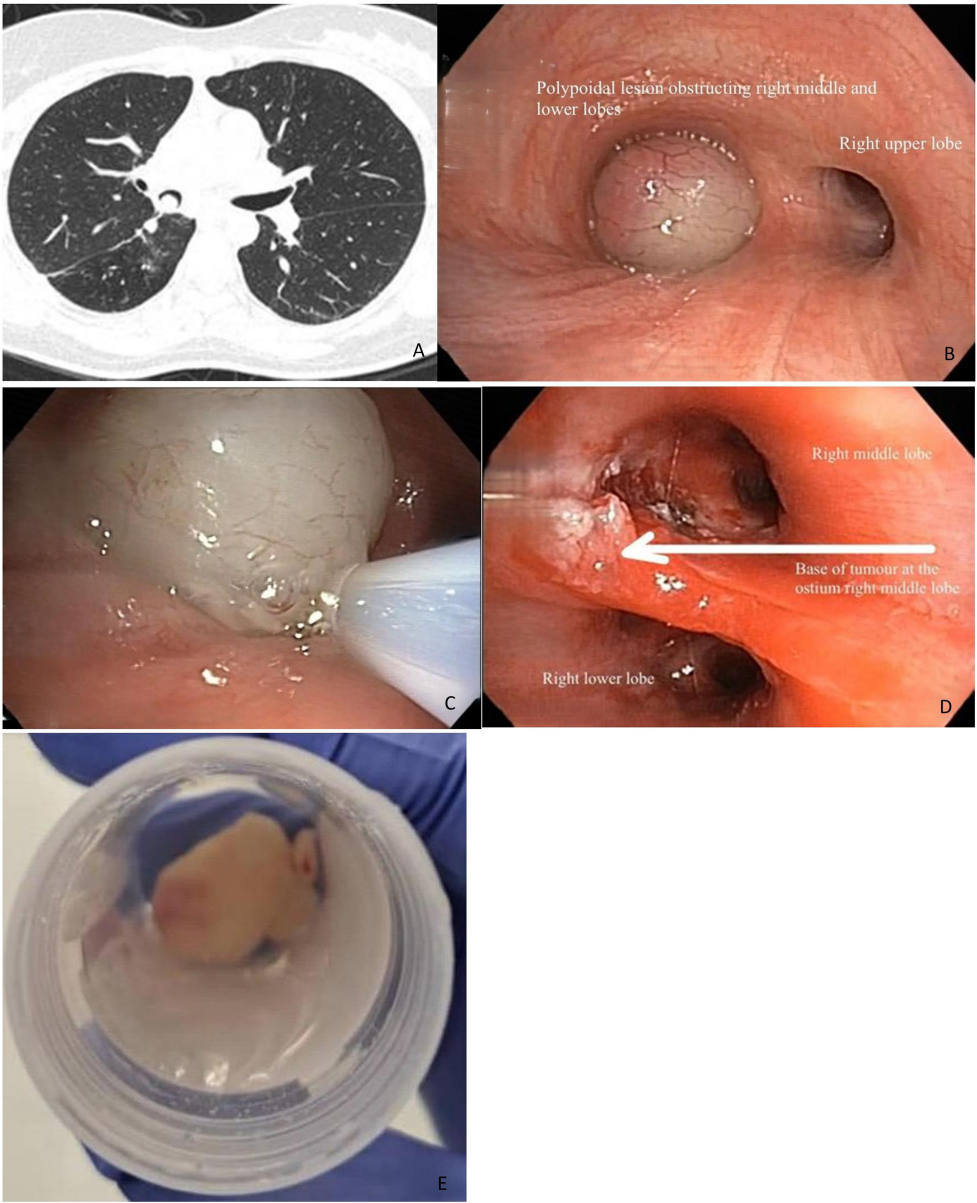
(A) CT showed right bronchus intermedius soft tissue density. (B) Bronchoscopic view of the polypoidal mass within the right bronchus intermedius. (C) Picture showing cryo‐resection. (D) Post‐cryo‐resection showing recanalisation of the right middle and right lower lobes bronchus. (E) Gross appearance of the mass after endobronchial electrocautery snare and cryoextraction.
